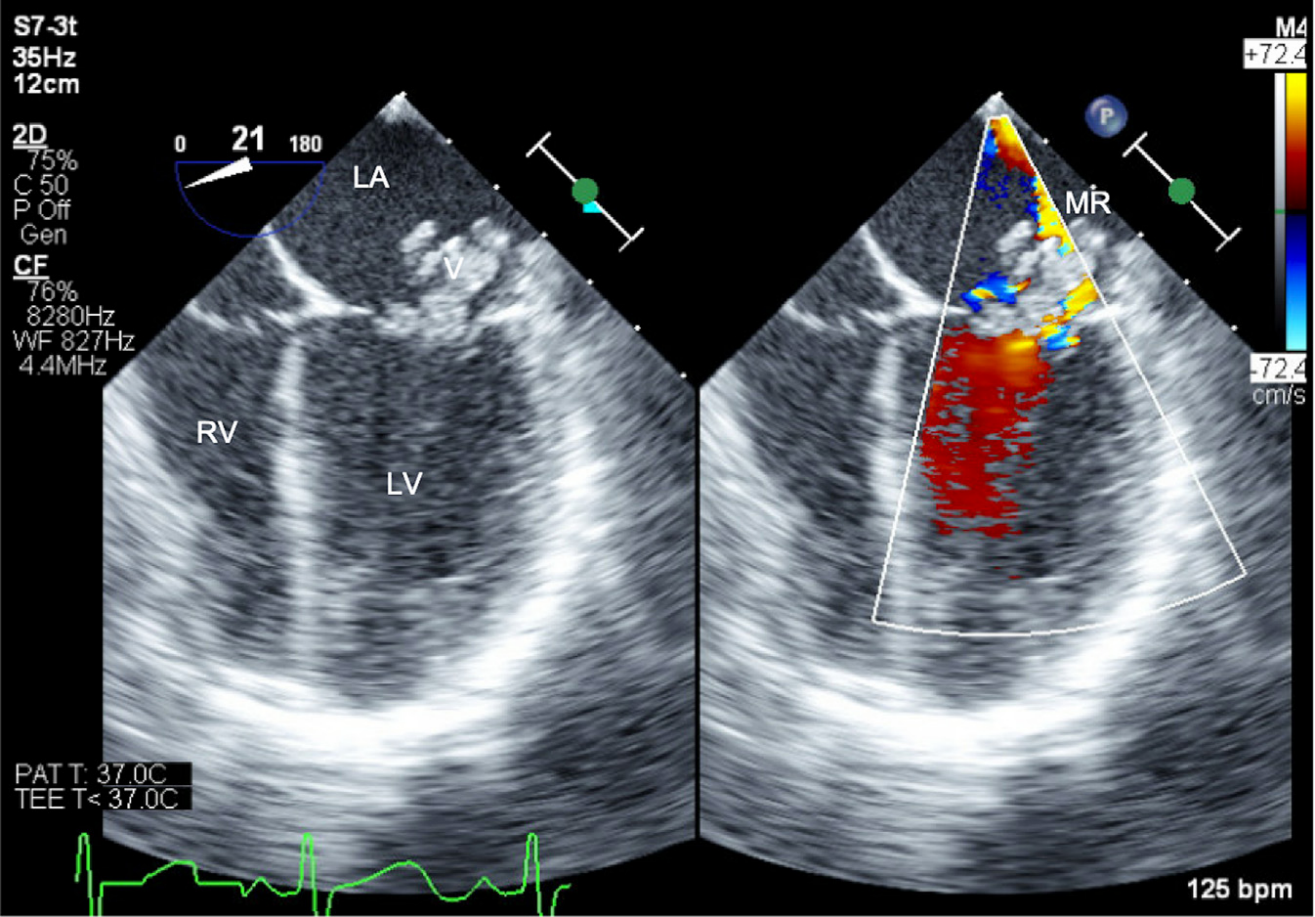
Transesophageal Echocardiogram of Vegetation Four-chamber view demonstrating a large vegetation on the mitral valve, with resultant mitral regurgitation (MR). Abbreviations as in Figure 1.
